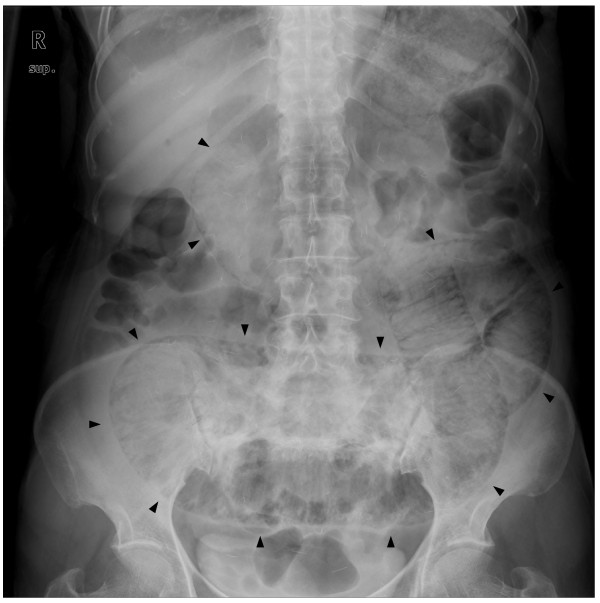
A plain abdominal radiograph. Abdominal X-ray showed a dilated colon and the presence of intraluminal air along the entire wall of the colon (black arrows).
